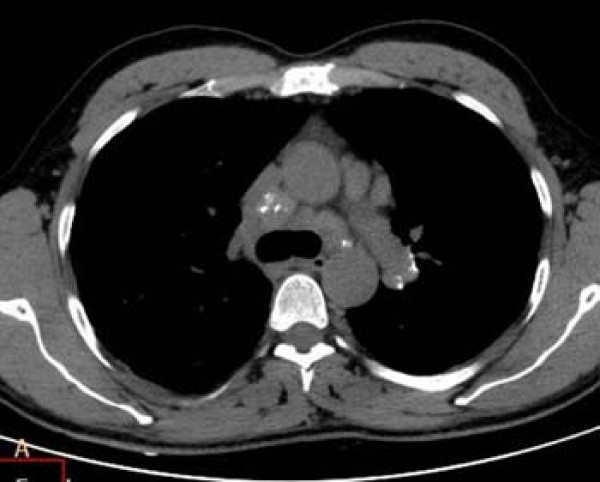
CT images of a 29-year-old man who worked as a denim sandblaster for 20 months. Paratracheal and left side hilar lymph nodes with central and eccentric calcification are shown.
